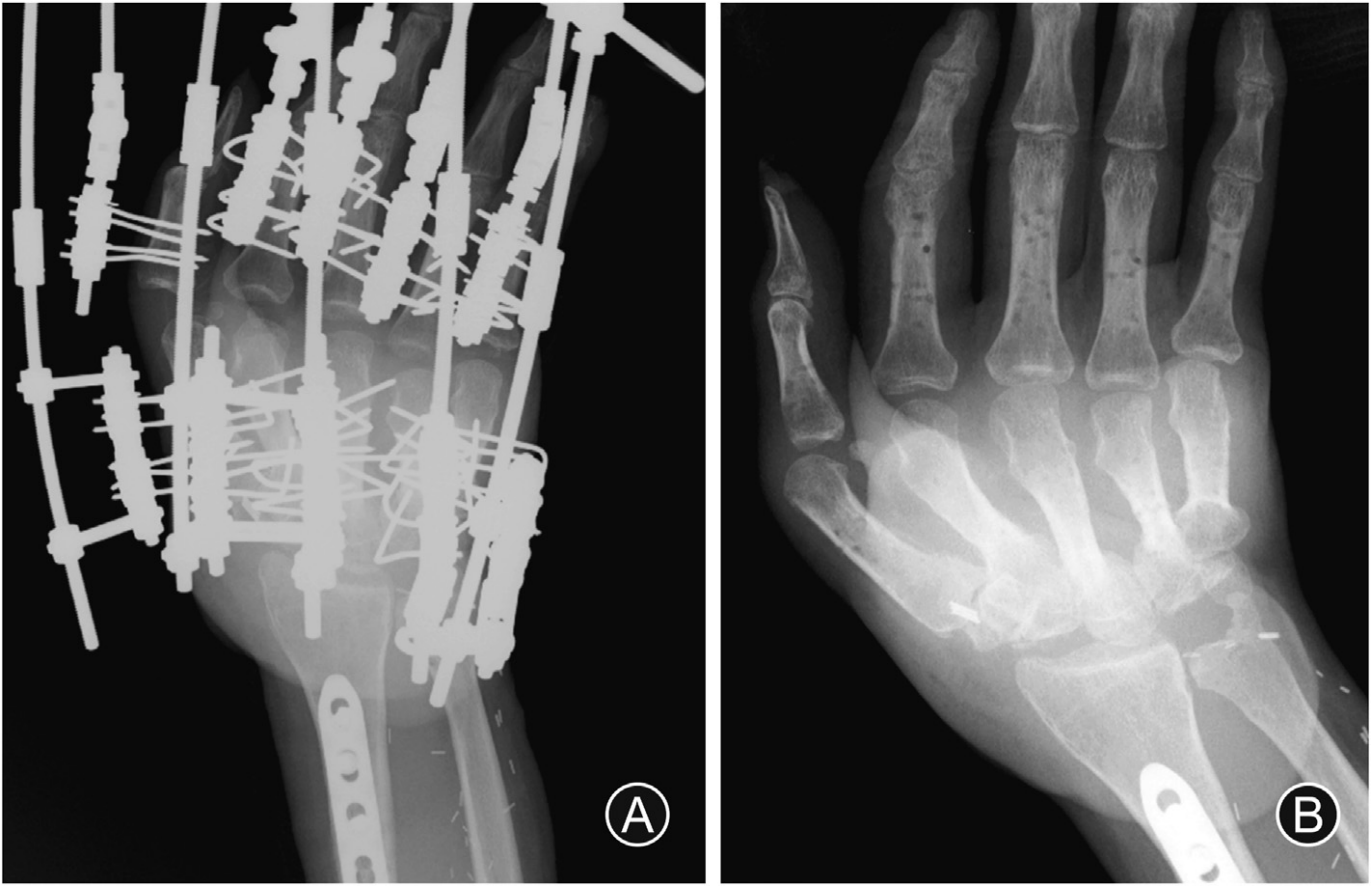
Radiograph showing contracture release (A: with an Ilizarov mini fixator, B: after external fixator removal).
